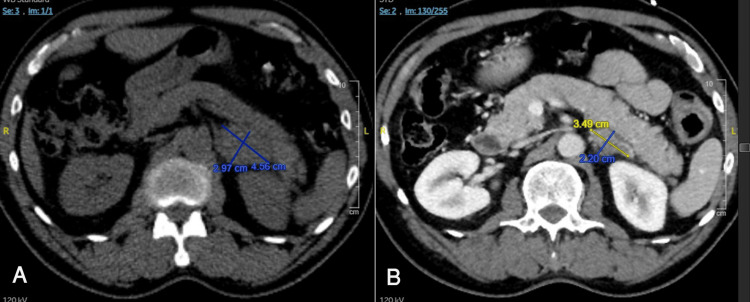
CT scan before (A) and two months after (B) treatment with capmatinib. Left adrenal mass size decreased from 4.56 x 2.97 cm to 3.49 x 2.20 cm. CT: computed tomography
